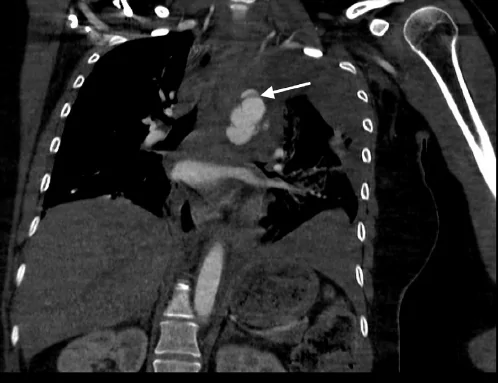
Computed tomography of the chest with contrast in coronal view demonstrating disruption of the descending thoracic aorta (arrow), consistent with blunt thoracic aortic injury.
